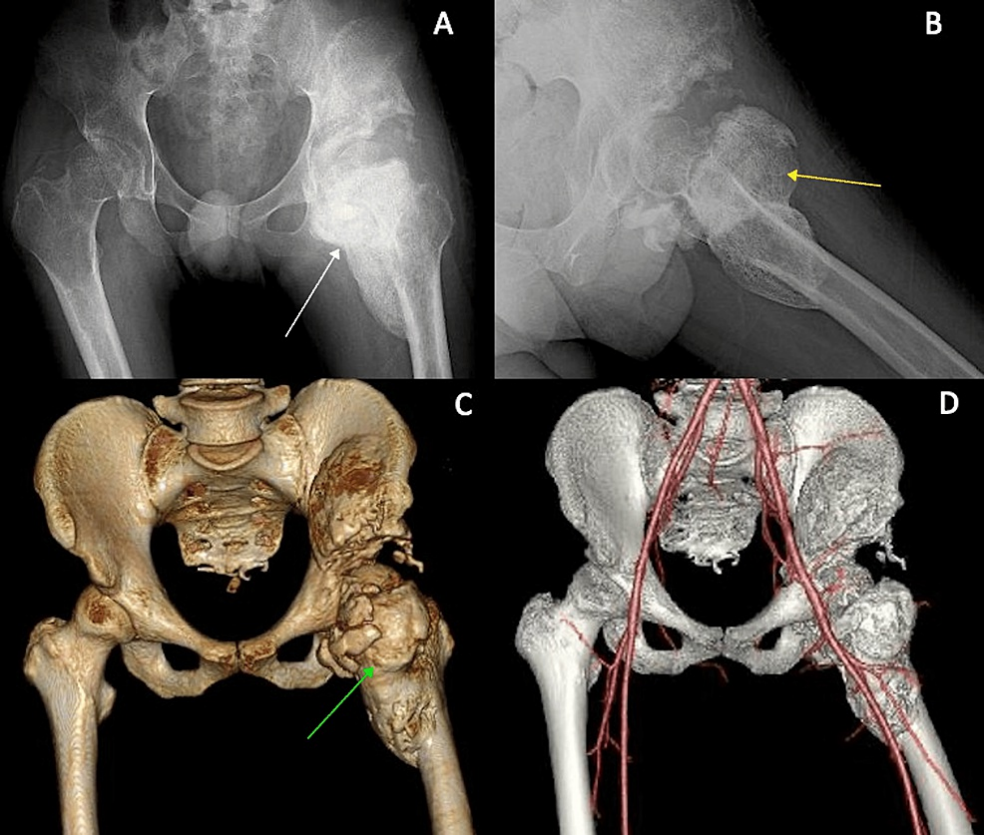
Patient 2 preoperative images Preoperative anteroposterior (A) and lateral X-ray (B), CT scan of the hip (C), and CT angiogram reconstruction of the vasculature (D). The white arrow depicts the wide extent of heterotopic ossification of the femur toward the medial aspect of the femur. The yellow arrow depicts the wide extent of heterotopic ossification of the femur. The green arrow depicts the wide extent of heterotopic ossification of the femur and erosion of the pelvis on CT scan. CT: computed tomography
